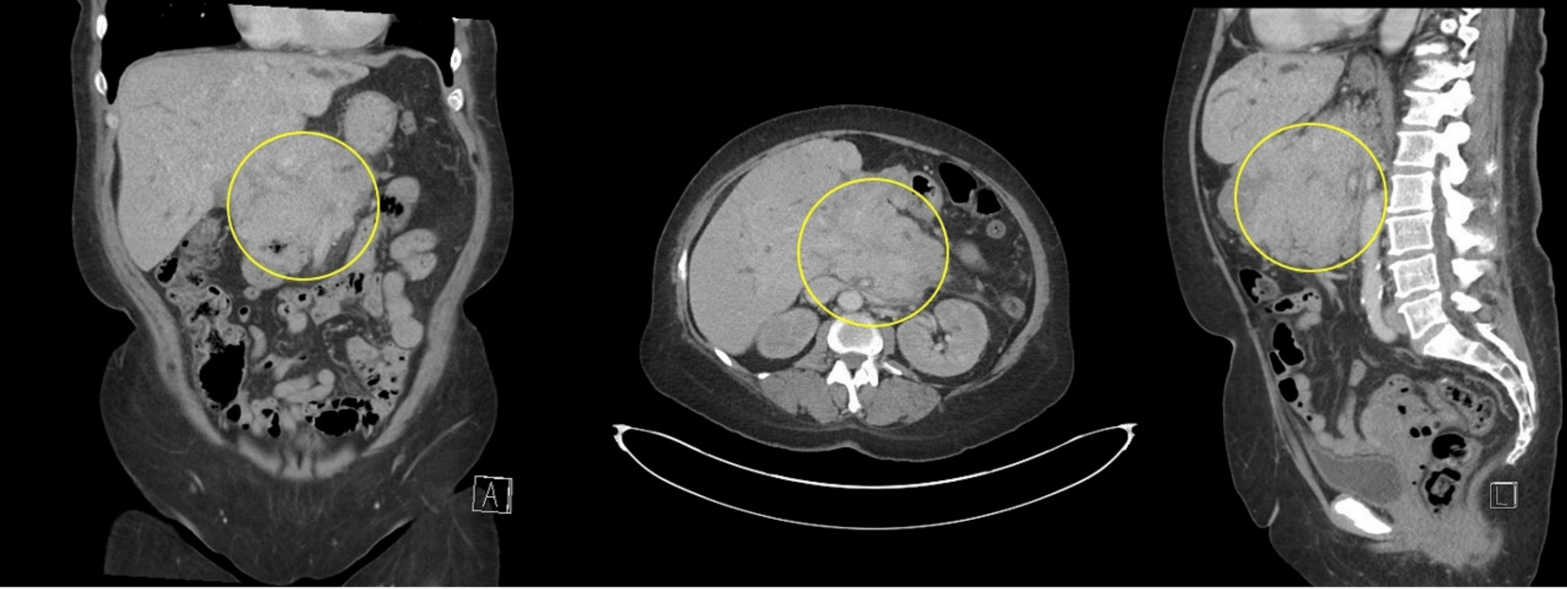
Coronal (left), axial (center), and sagittal (right) contrast-enhanced CT of the abdomen Coronal (left), axial (center), and sagittal (right) contrast-enhanced CT images of the abdomen show a large, lobulated soft-tissue mass (circled), replacing the majority of the pancreatic head and body. The lesion measures approximately 12.1 × 9.0 × 7.9 cm, encases the SMA and portal vein, and extends toward the region of the gallbladder fossa. Note the minimal prominence of the pancreatic duct within the mass.​ CT, Computed Tomography; SMA, Superior Mesenteric Artery
